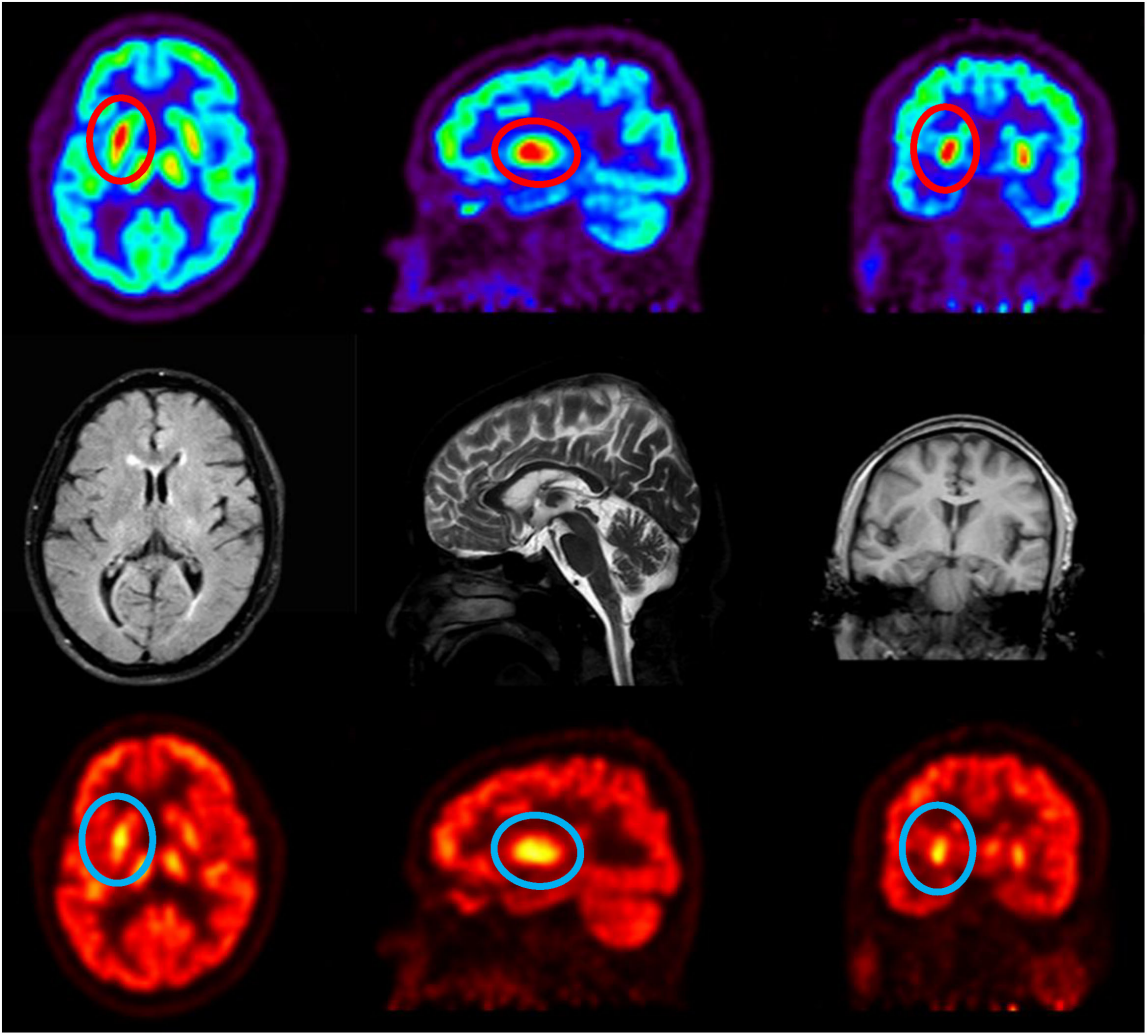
18F-FDG PET/CT reveals metabolic alterations, enhancing diagnostic accuracy. The radioactive tracer 18F-FDG evaluates tissue metabolic activity, revealing markedly elevated metabolism in the right basal ganglia relative to the left.
